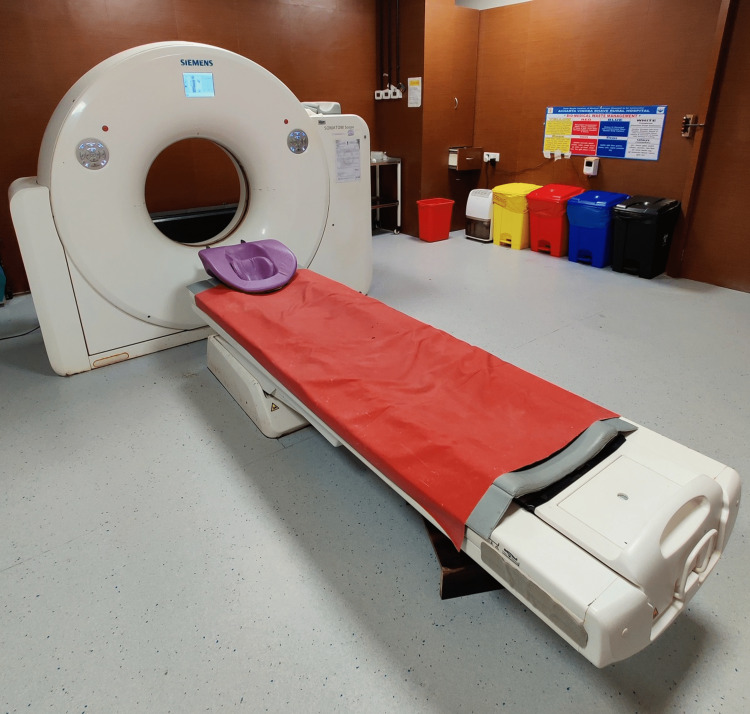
CT machine Image credit: Harsh Lahoti
